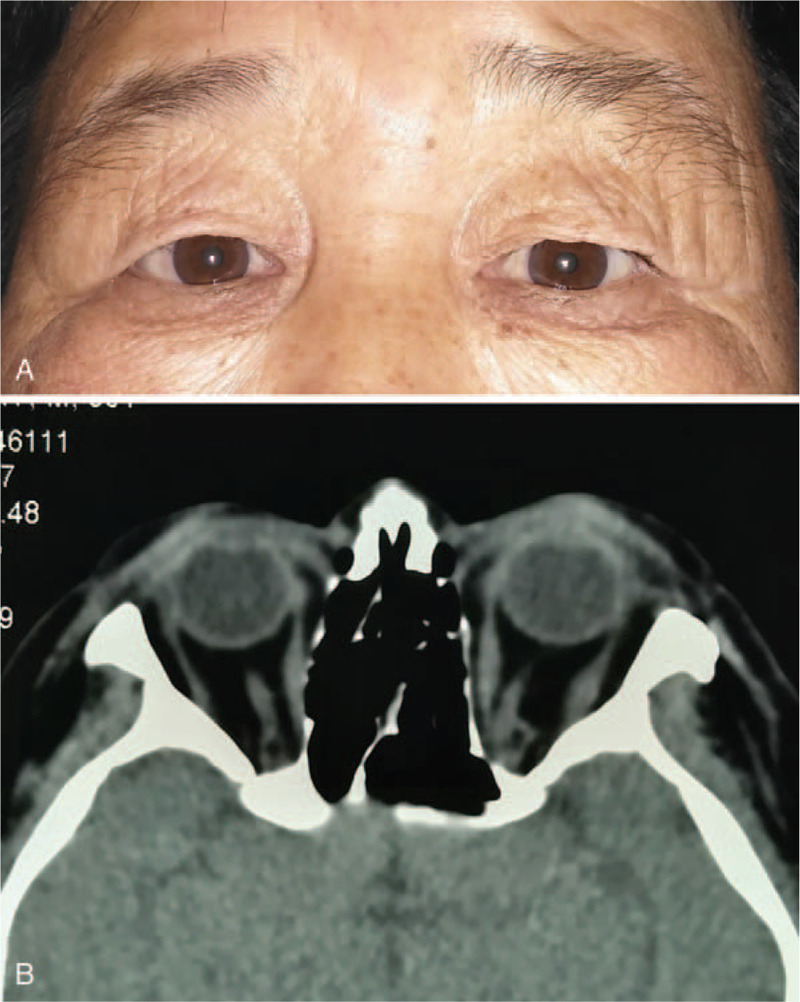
28 months after the surgery. (A) No abnormalities were observed in the patient. (B) The orbital CT scans showed the orbit was normal, and no significant lesions were observed.
